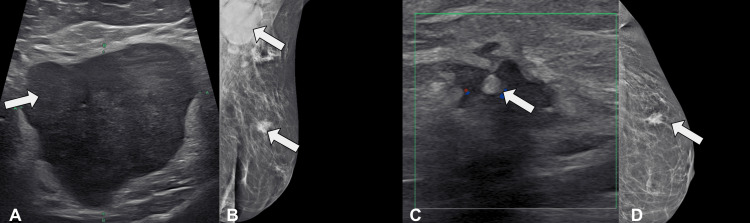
Initial diagnostic imaging of the left breast and axilla. (A) Axillary ultrasound showing an enlarged hypoechoic lymph node with irregular borders (arrowhead). (B) Mammogram showing a spiculated breast mass and enlarged axillary lymph node (arrowheads). (C) Breast ultrasound showing an irregular hypoechoic mass with posterior acoustic shadowing (arrowhead). (D) Craniocaudal mammogram showing a spiculated mass in the upper outer quadrant (arrowhead).
